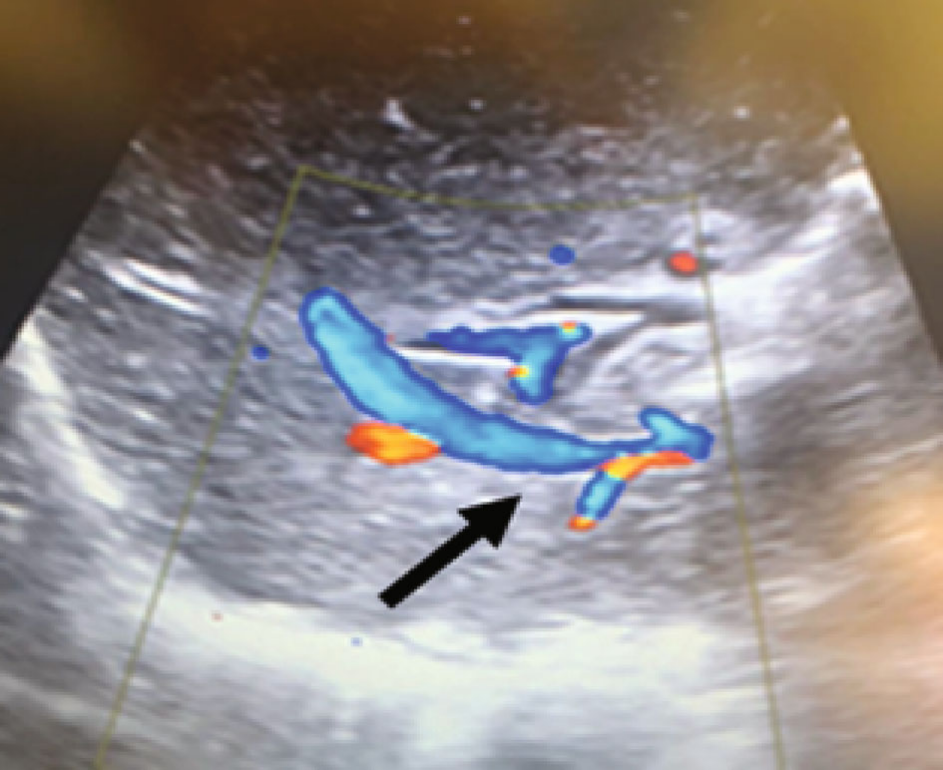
Intraoperative Doppler ultrasonography showing IRHV patency after resection, with hepatofugal flow (arrow).
